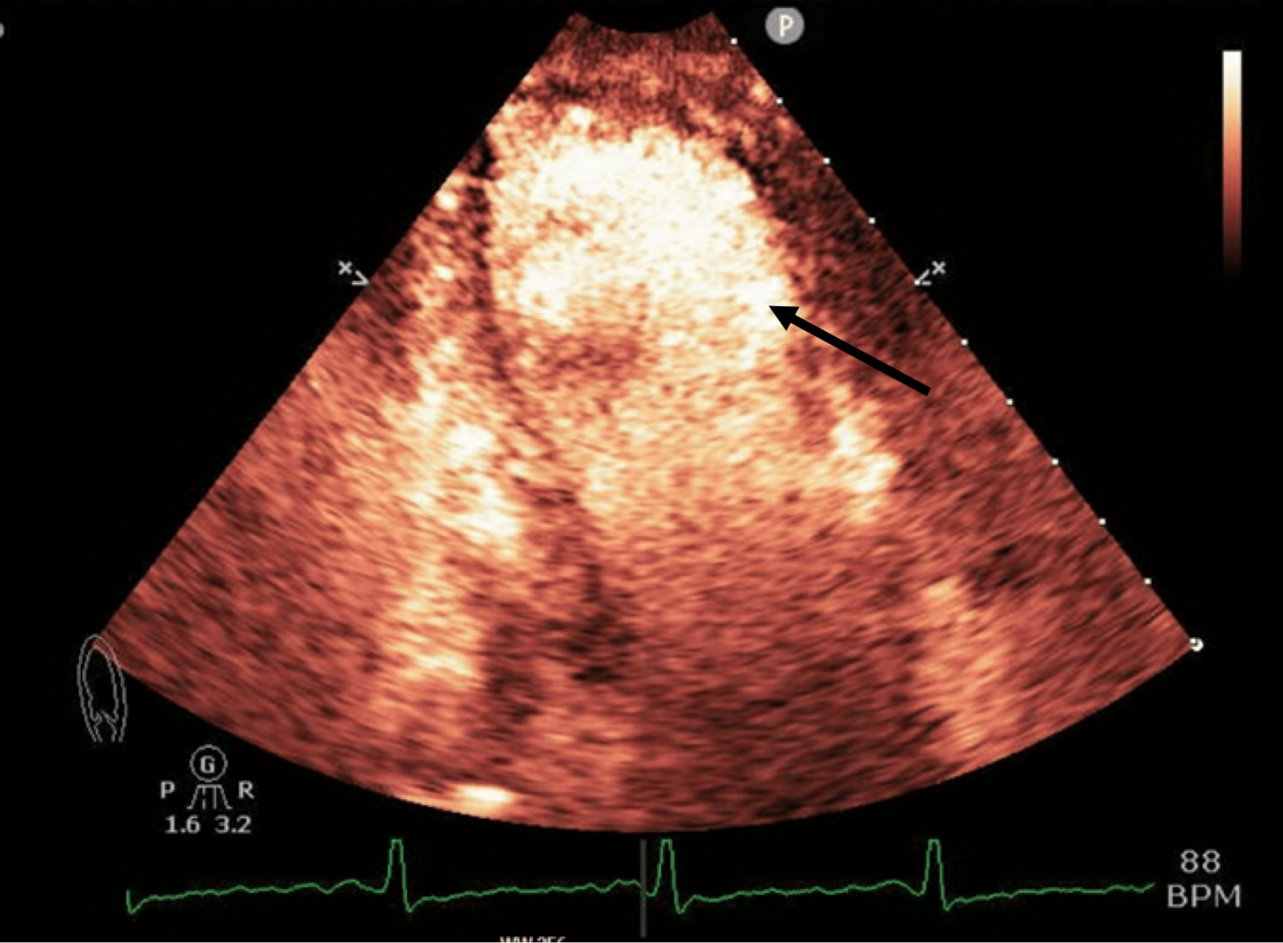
Two-chamber echocardiograph demonstrating ballooning of the basal segment at the end of systole indicated by black arrow.
